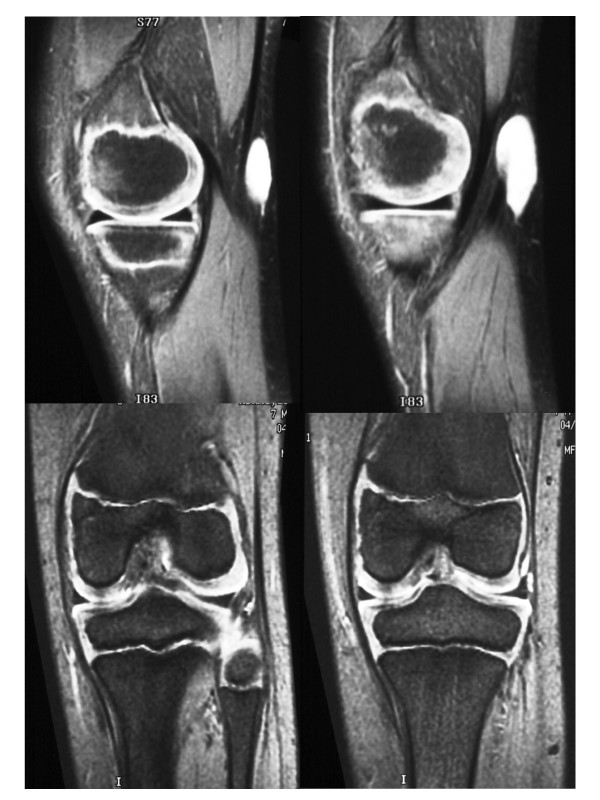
MRI at diagnosis (case one).
